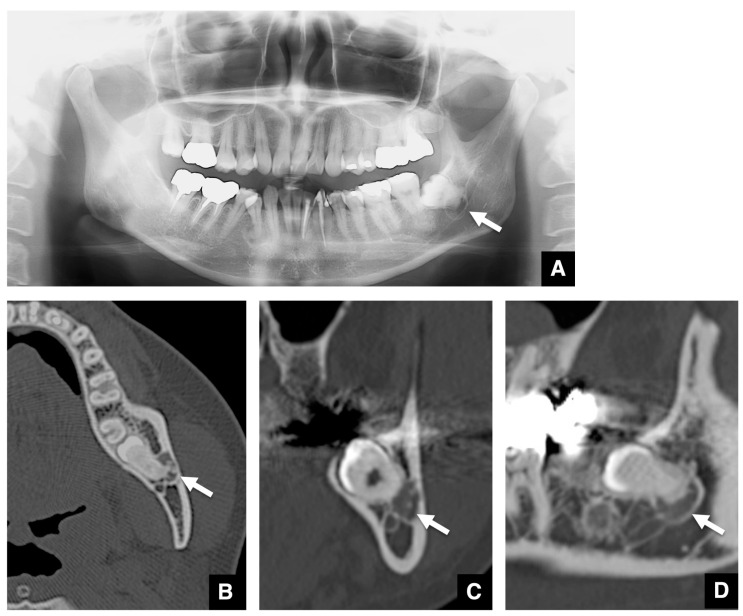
Representative images of COD related to an embedded mandibular third molar with no radiopaque structure identified on the panoramic radiograph. (A) The COD related to an embedded mandibular third molar is visualized as a round area (arrow) with a radiolucent area in the periapical region on the panoramic radiograph. The sclerotic rim is clear, and no radiopaque structure is seen. (B) The COD related to an embedded mandibular third molar is visualized as a round area (arrow) with low density in the periapical region of the affected tooth on axial MDCT. The low-density mass contains high-density, dot-like structures. The high-density structures are partially contiguous with the roots of the mandibular third molar. There is thinning of the cortical bone adjacent to the lesion. (C) The mass (arrow) and internal high-density structures are shown on coronal MDCT. (D) The mass (arrow) and internal high-density structures are shown on sagittal MDCT.
